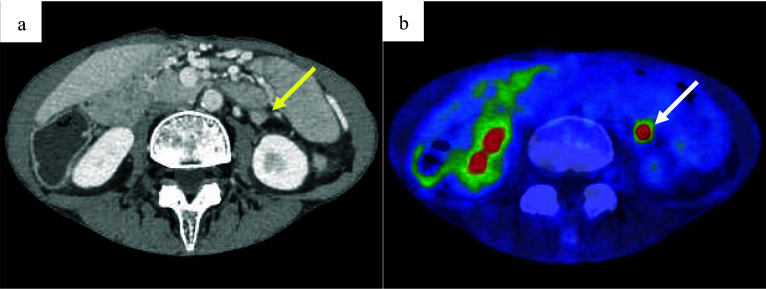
Follow-up contrast-enhanced computed tomography (CT) and positron emission tomography (PET)/CT 2 years after the initial surgery. a Contrast-enhanced CT shows a 10 mm mass (arrow) adjacent to the inferior mesenteric artery. b PET/CT scan reveals a mass (arrow) at the same location with elevated fluorodeoxyglucose uptake, indicated by a maximum standardized uptake value of 10.6
